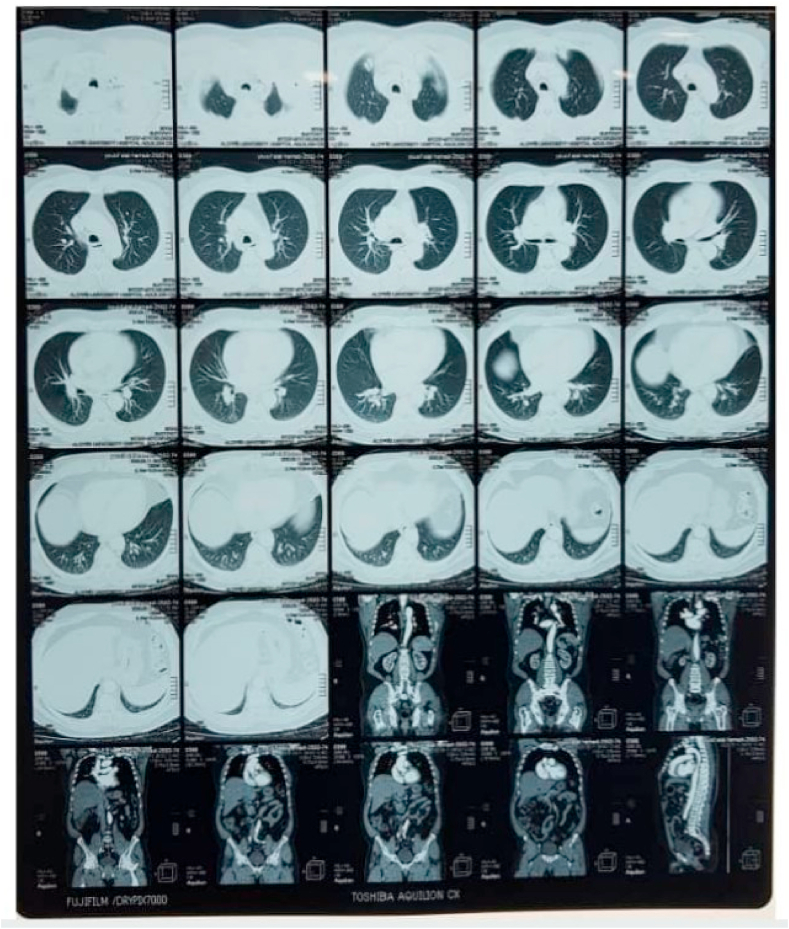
CT with contrast of Chest, abdomen, and pelvis shows normal findings.
